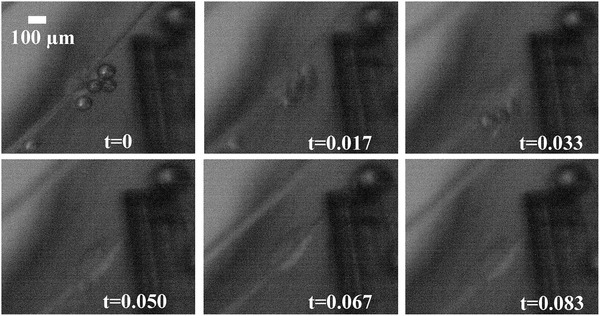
Longitudinal collage showing cavitation on cell‐laden microfibers. The samples are in the PBS tank and pictures are taken with high‐speed camera and telescopic lens. At *t* = 0 the ultrasonic transducer is activated. The later frames show the dramatic oscillation and collapse of MBs in response to ultrasound exposure.
